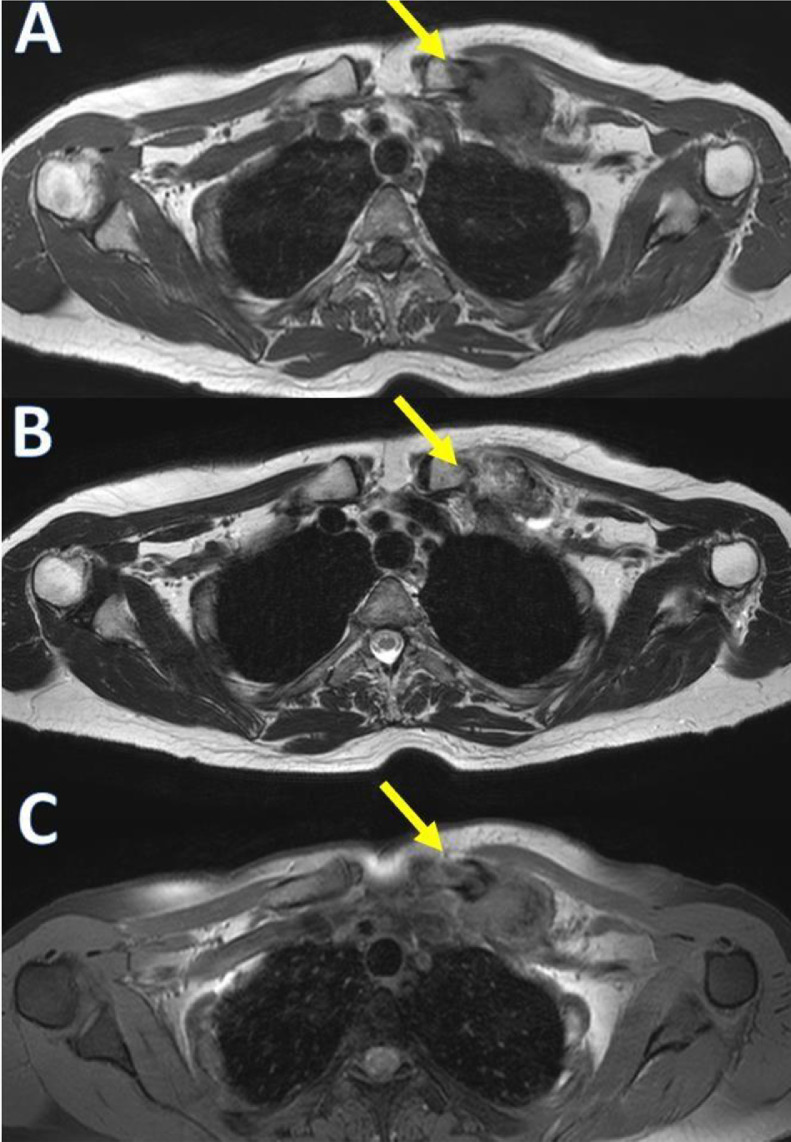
Magnetic resonance imaging (MRI) using T1 (A), T2 (B), and T1 Fat Sat (C) weighted sequences in the axial plane indicate a soft tissue lesion predominantly isointense or slightly hyperintense, relative to adjacent muscles in T1-weighted sequence and predominantly hyperintense in T2-weighted sequences (yellow arrows). Hypointense marginal portion, due to the presence of calcifications, is observed in both T1 and T2 weighted images (green arrows).
